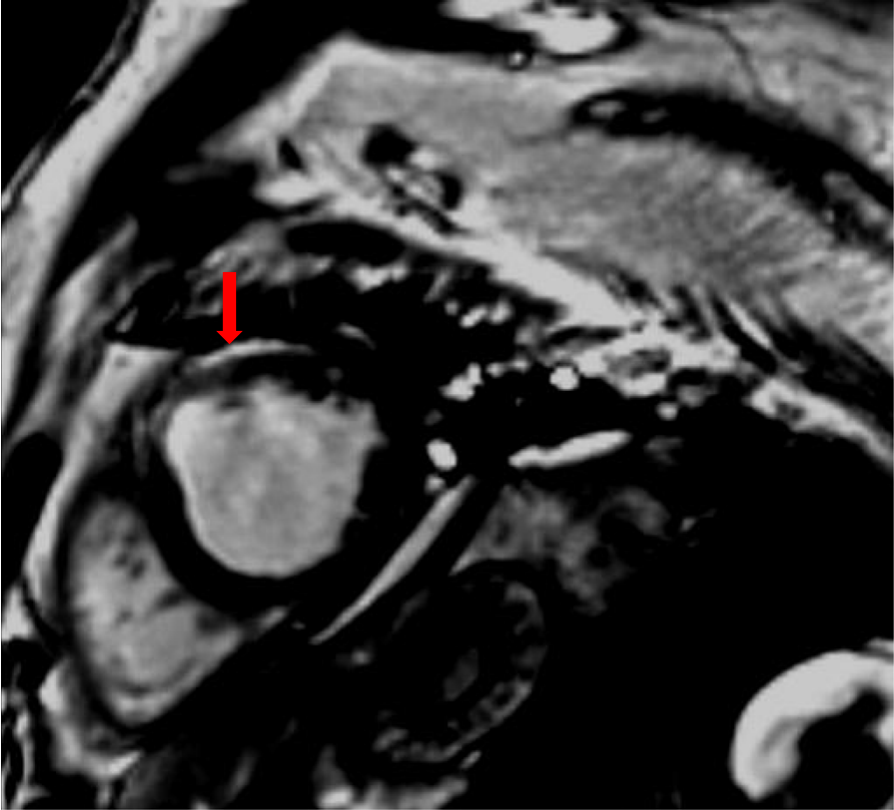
Cardiac magnetic resonance imaging. Cardiac MRI revealed thinning of apical anterior wall of left ventricle and subendocardial late gadolinium enhancement (*arrow*); estimated left ventricular EF was 44.3 %
